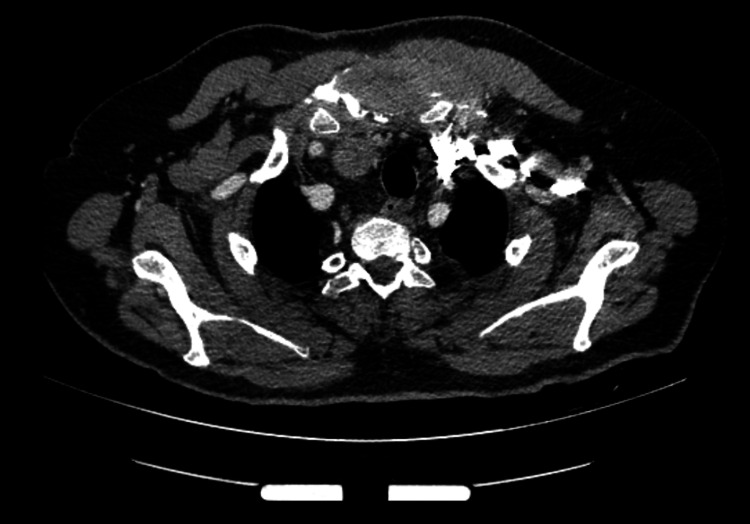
CT revealed a massive 4.7 × 4.6 × 4.5 cm sternal mass. CT: computed tomography.
